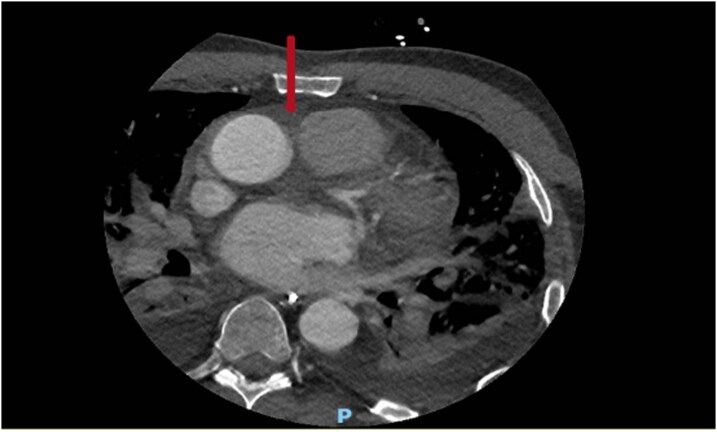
CT aorta demonstrating an aortic root abscess.
